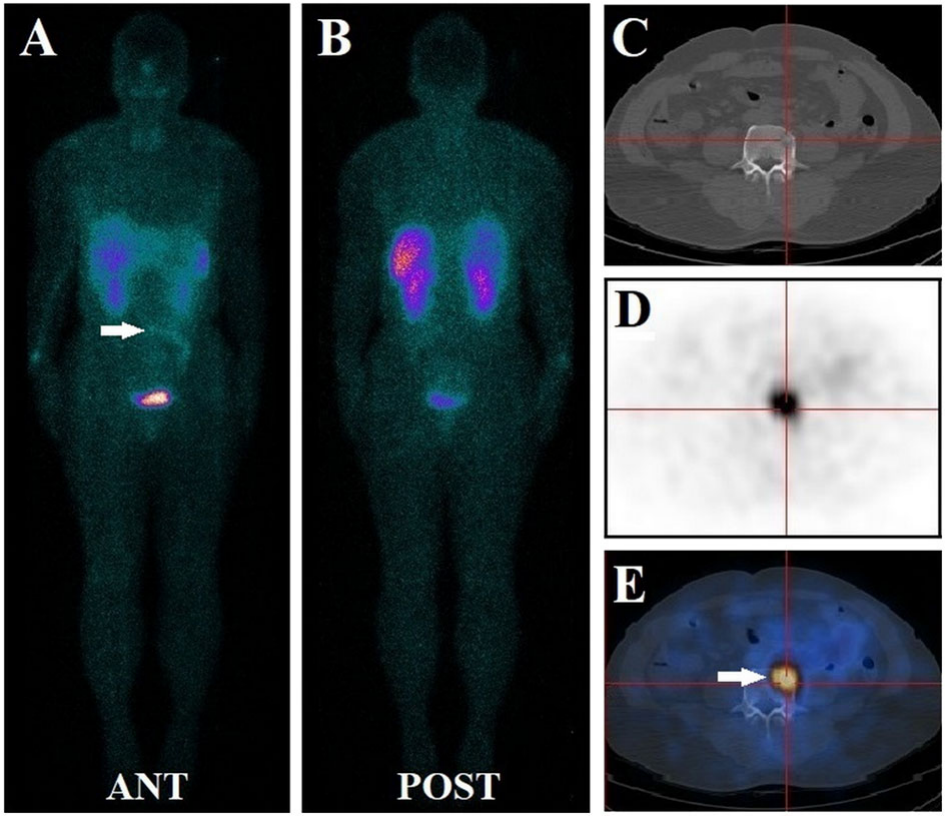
A 72-year-old male had diffuse bone pain and muscle weakness for nine years. Before this study, his serum phosphate level was lower than normal at 0.65 mmol/L. the patient underwent a ^99m^Tc-HYNIC-TOC whole-body planar scan, and no evident focus of elevated activity was identified (A, B). Subsequent thoracic and abdominal SPECT/CT images revealed small ^99m^Tc-HYNIC-TOC positive foci in the fourth lumbar vertebra (white arrow) (C–E). The foci (white arrow) were missed during planar scanning due to radio-retention interference from adjacent bowels. The diagnosis of phosphaturic mesenchymal tumor was confirmed after the lesion was surgically removed. The long-term symptoms of this patient disappeared promptly, and his post-surgical serum level increased to normal at 1.21 mmol/L.
